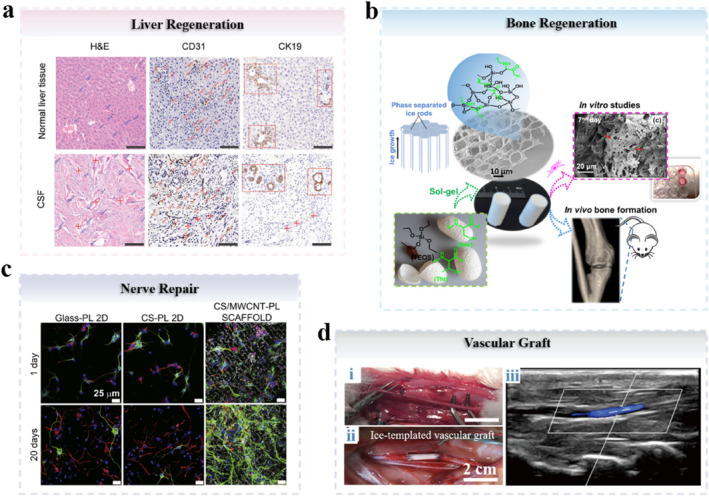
Examples of ice‐templated scaffolds for various tissue engineering applications. (a) Ice‐templated chitosan‐fibers cryogel (CSF) for in situ liver regeneration [147]. (b) Ice‐templated silica‐silk fibroin bioaerogel for in vivo bone formation [148]. (c) Ice‐templated chondroitin sulphate/MWCNT scaffold for embryonic neural progenitor cell differentiation [149]. (d) Ice‐templated vascular graft for revasculature [150]. Optical images of (i) Native vessel and (ii) ice‐templated vascular grafts in rabbits. (iii) In situ sonographic images of ice‐templated vascular grafts. (a) Adapted with permission [147]. Copyright 2023, Wiley‐VCH. (b) Adapted with permission [148]. Copyright 2019, American Chemical Society. (c) Adapted with permission [149]. Copyright 2014, Elsevier. (d) Adapted with permission [150]. Copyright 2019, American Chemical Society.
